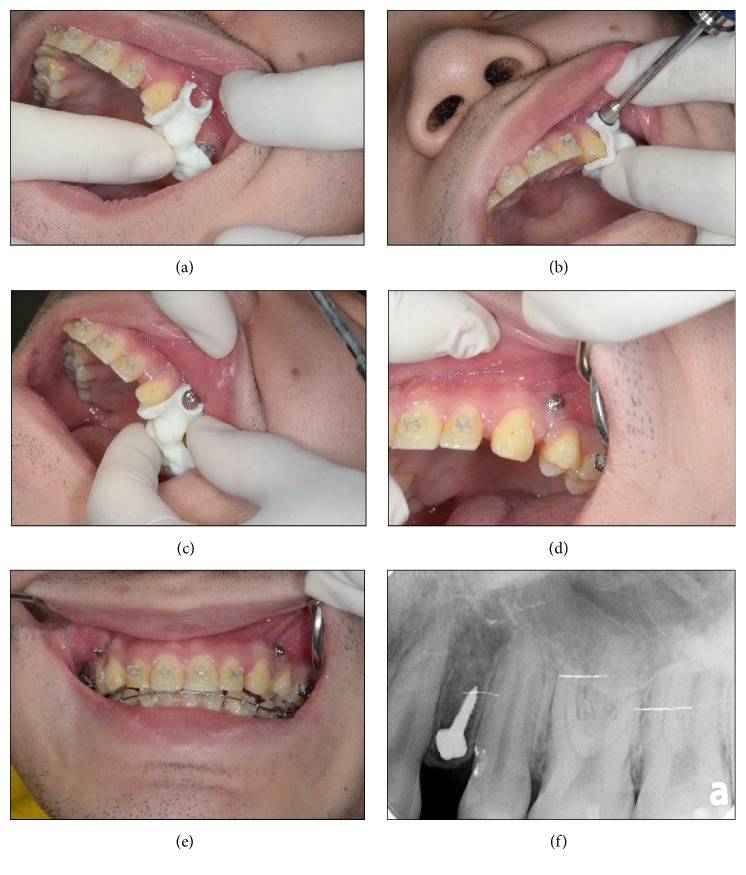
(a) The surgical templates were placed intraorally onto the teeth by a clinician; (b) to (e) showed the clinical application of the customized surgical template; (f) X-rays were taken after implantation to assess whether damage to the peripheral tissue occurred for assessing miniscrew stability.
